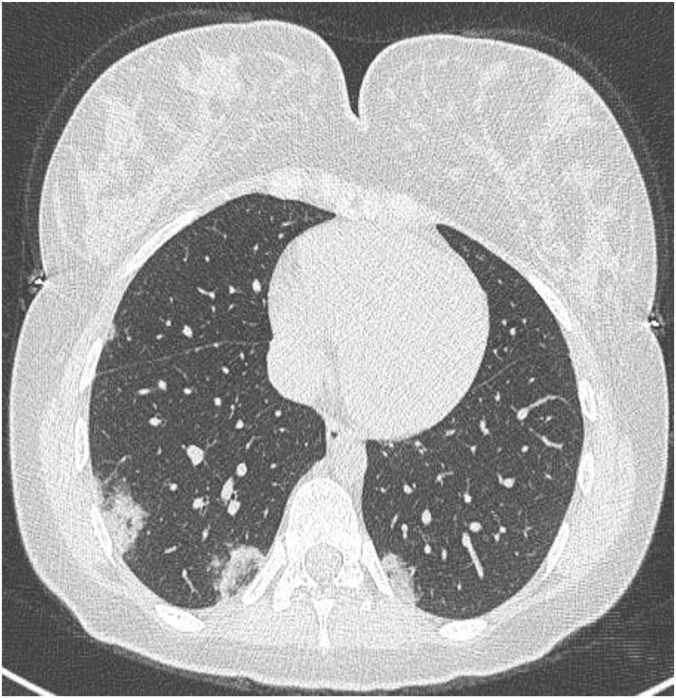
Axial CT image showing bilateral posterior, peripheral, and rounded ground glass opacities in a patient with organizing pneumonia secondary to dermatomyositis; typical Covid-19 imaging features.
